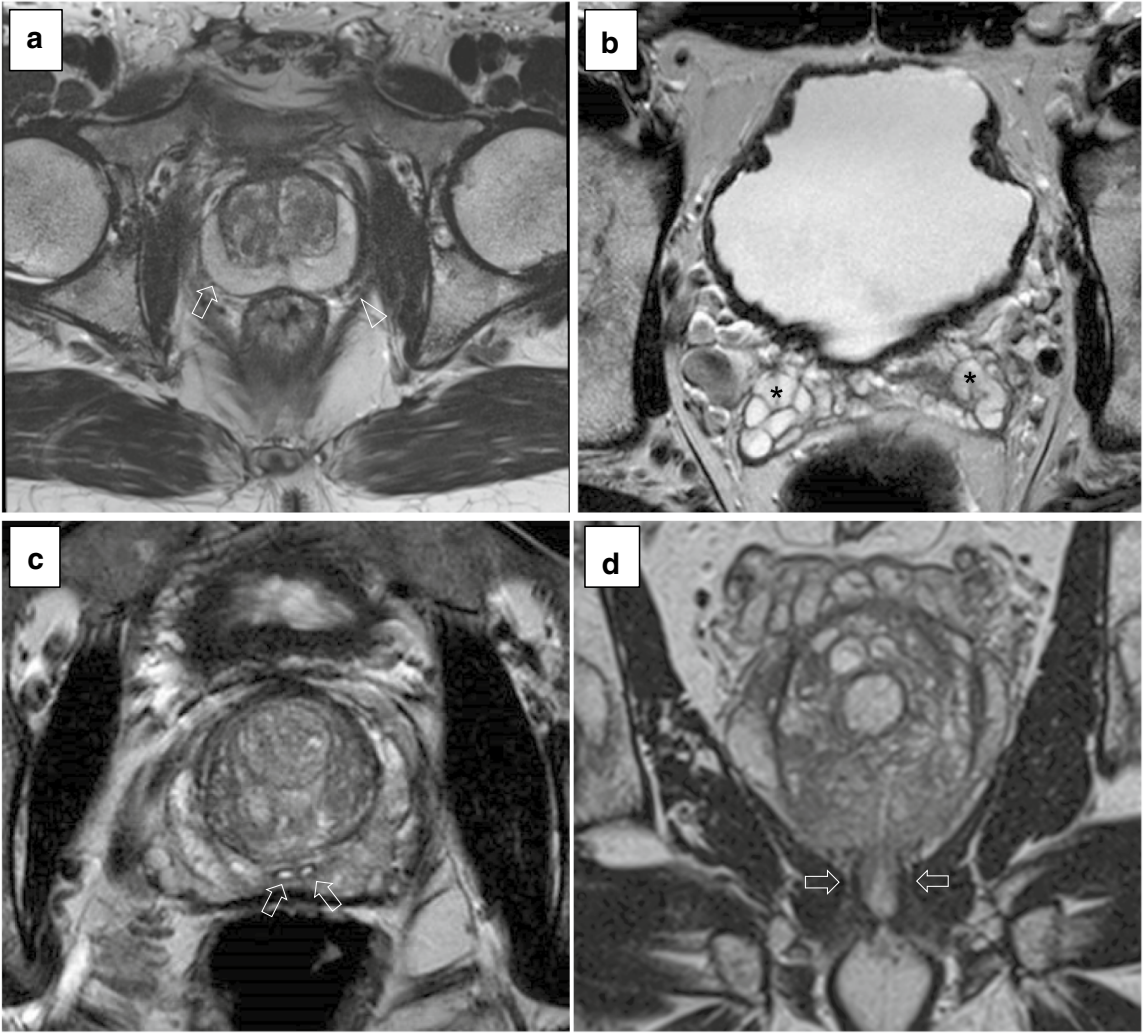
Axial (a–c) and coronal (d) T2-weighted images of good quality showing the prostatic capsule (a, arrow), the neurovascular bundle (a, arrowhead), the seminal vesicles (b, asterisks), the ejaculatory ducts (c, arrows) and the external sphincter (d, arrows), as mentioned in the visual assessment of the PI-QUAL scoring sheet
